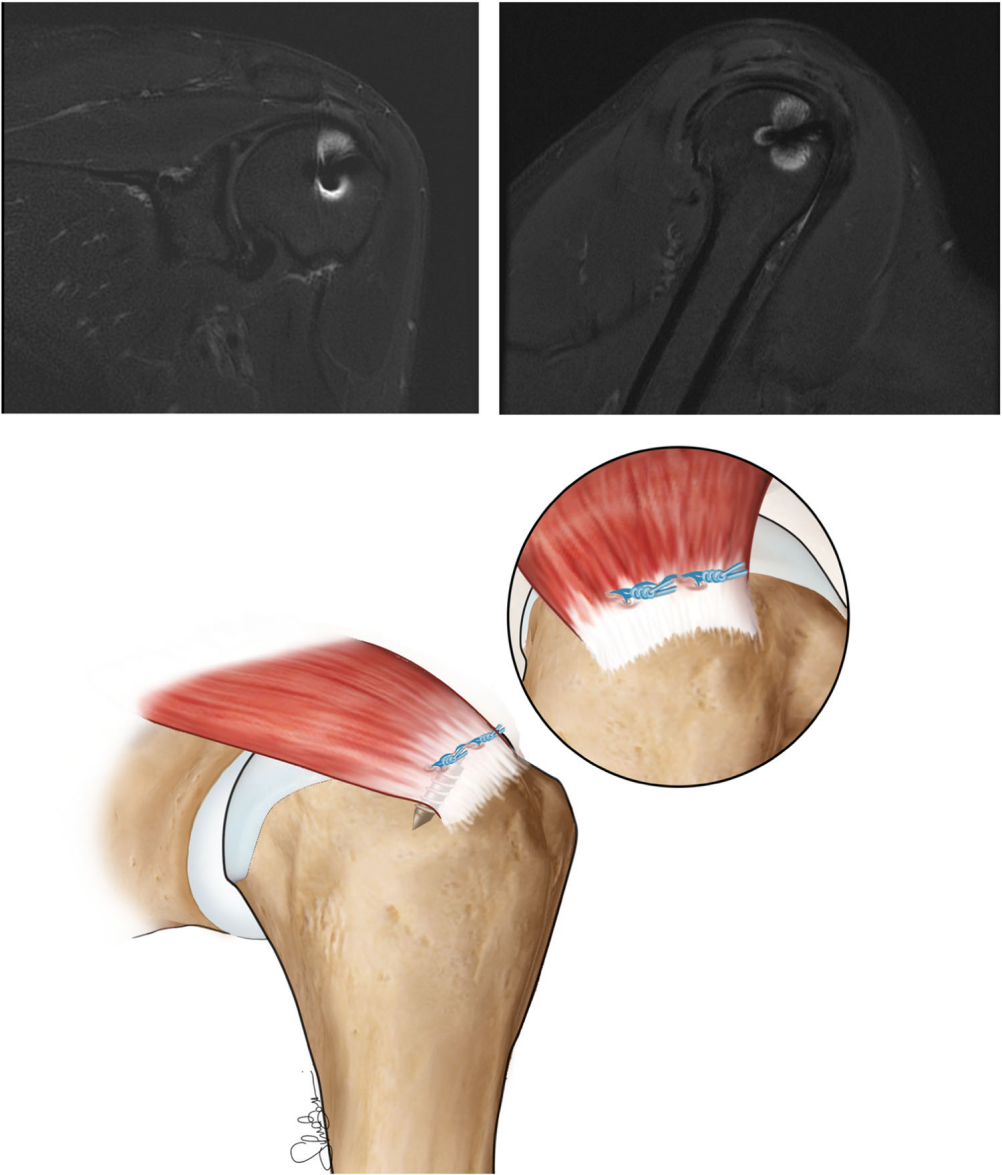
Single‐row technique at 24 months minimum follow‐up. 3‐T MRI images show the supraspinatus tendon reattached to its footprint using one Corkscrew 5.5 mm metallic anchors preloaded with No. 2 Fiberwire sutures (Arthrex). The tendon appears continuous and well‐integrated. MRI, magnetic resonance imaging.
